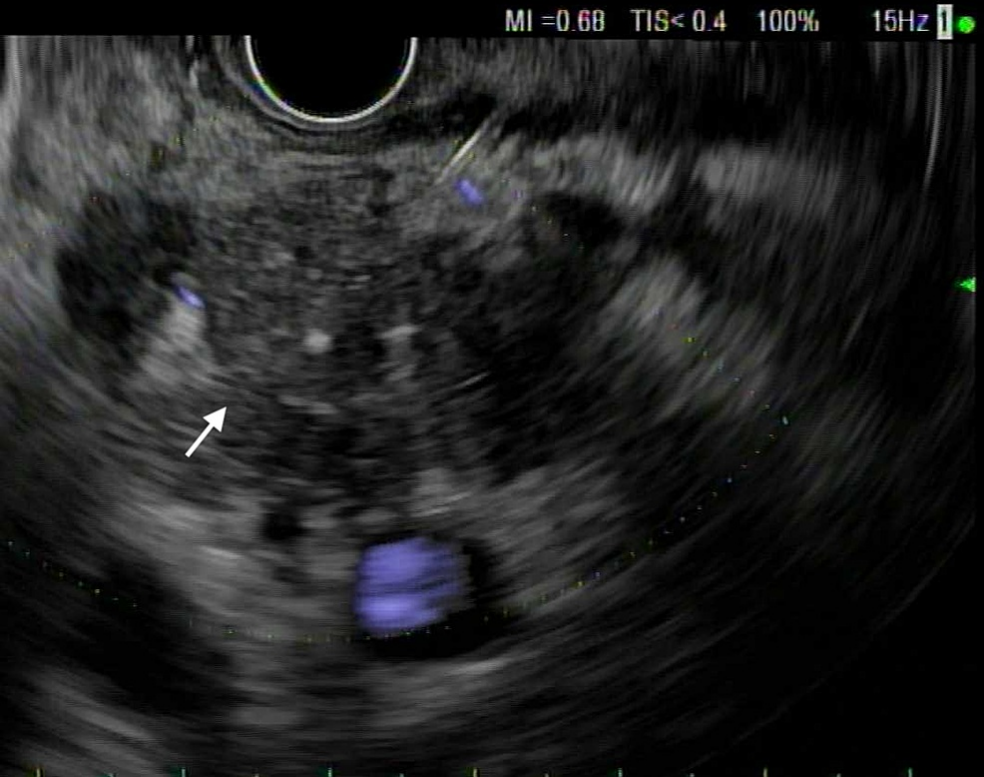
Pancreatic carcinoma seen on endoscopic ultrasound (EUS)
